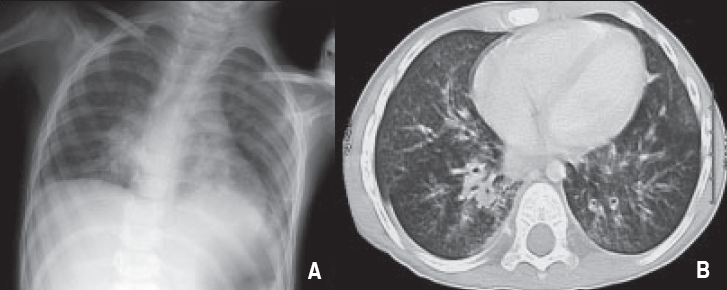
A patient with seizure, brain atrophy, swallowing incoordination, Gastroesophgeal reflux and history of recurrent aspiration pneumonia/ Gastrostomy tube feeding. A. Chest X-ray: Diffuse airspace disease with infiltrates seen mainly in Peri hilar and basal parts. B. CT chest: Extensive bronchial wall thickening with peribronchial inflammatory changes throughout both lungs. peribronchial infiltrate in RLL and LLL with dilated bronchi in both lobes.
